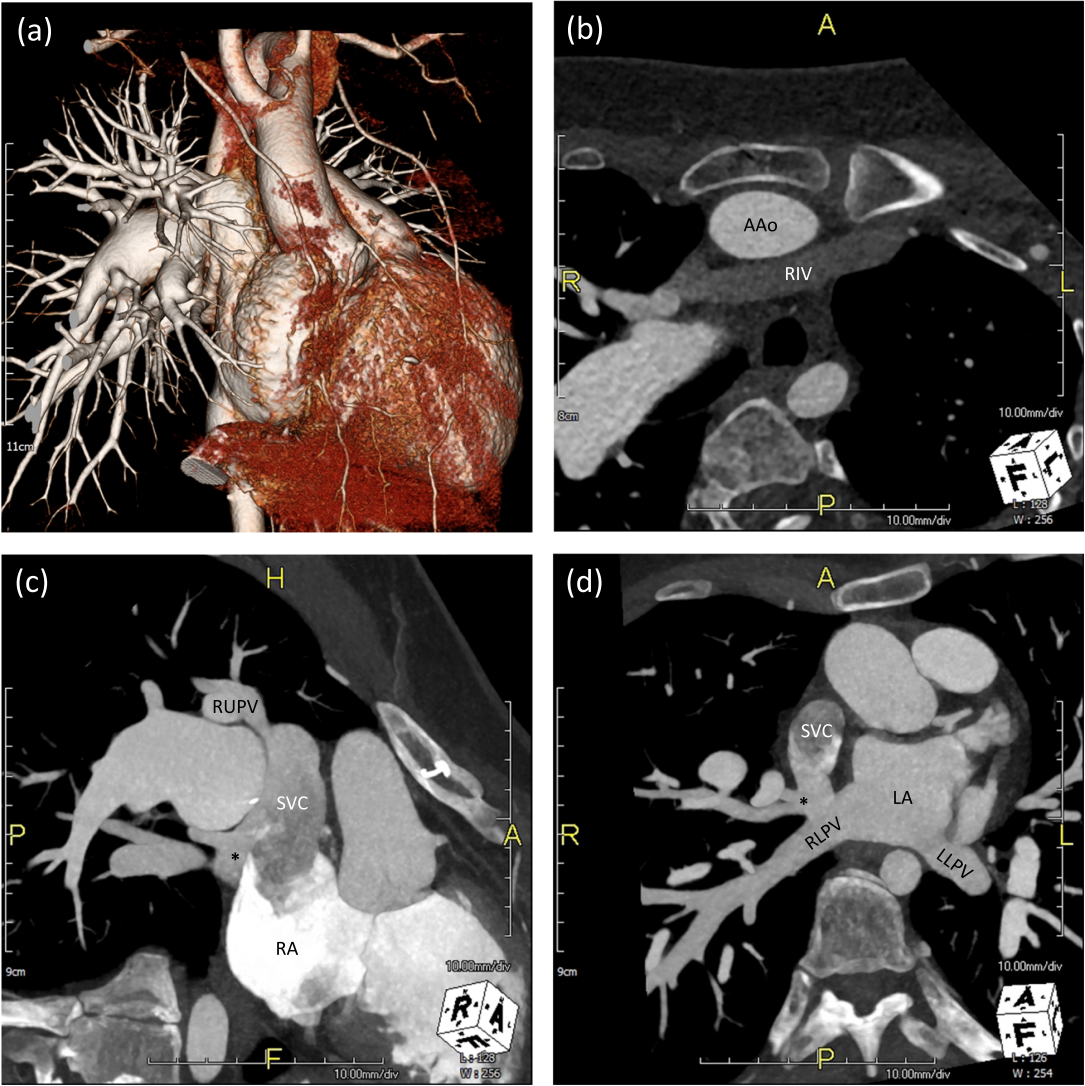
Preoperative chest computed tomography with (a) 3-dimensional reconstruction. Computed tomography demonstrating (b) RIV, (c) anomalous connection of RUPV and RMPV (asterisk) to SVC, and (d) dual connection of RMPV (asterisk) to SVC and LA. AAo, ascending aorta; RIV, retroaortic innominate vein; RUPV, right upper pulmonary vein; RMPV (asterisk), right middle pulmonary vein; SVC, superior vena cava; RA, right atrium; LA, left atrium; RLPV, right lower pulmonary vein, LLPV, left lower pulmonary vein
